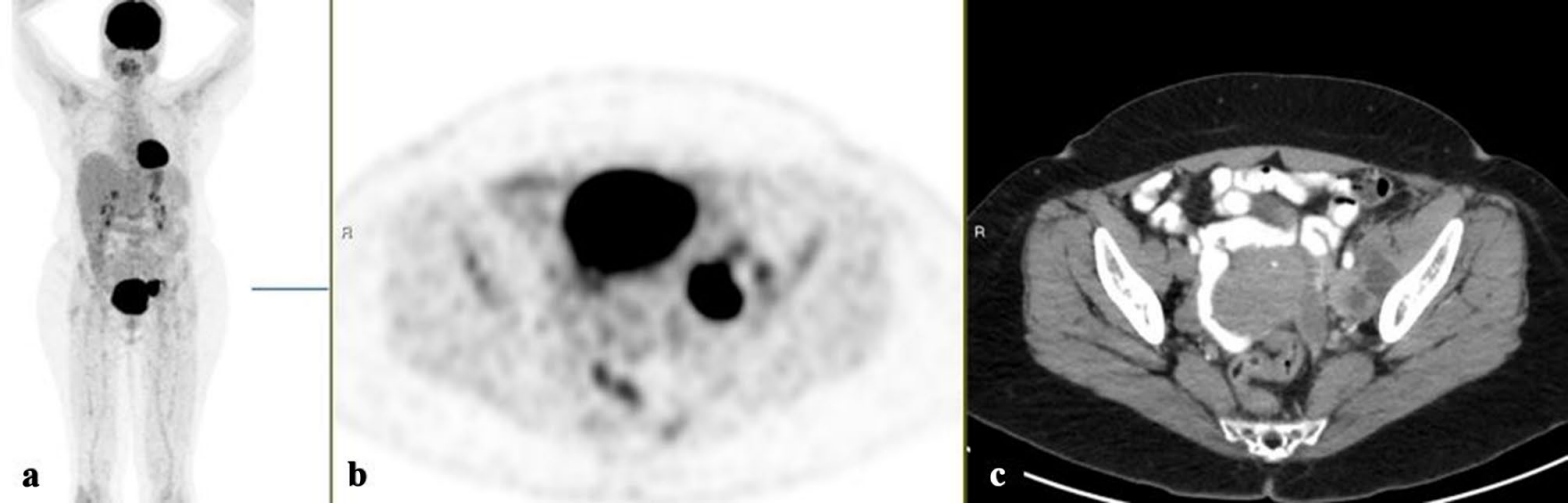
[18F]FDG-PET/CT: maximum intensity projection (MIP), (a) representative PET (b) and CT (c) axial slices. A 65-year-old woman with a biopsy-proven squamous cell carcinoma from a pelvic mass (A. b). Left obturator [18F]FDG avid enlarged lymph nodes.
